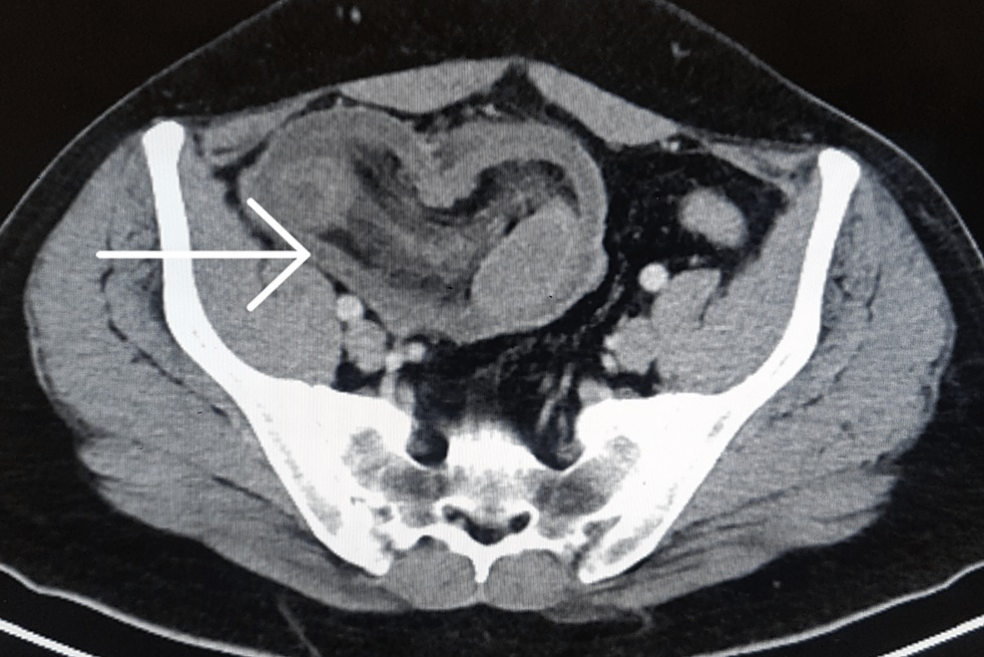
Contrast-enhanced CT of the abdomen and pelvis showing the ileocolic intussusception.
